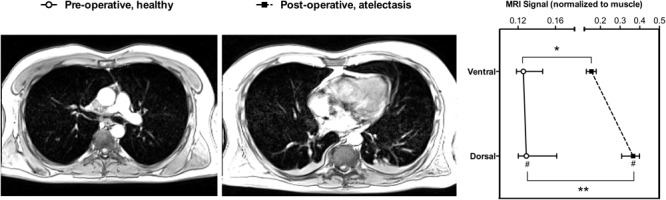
Magnetic resonance imaging quantitative analysis in a representative patient. Pre-operative scan with healthy lungs (Left), and post-operative scan showing atelectasis (Middle). (Right) Shows the lung MRI signal intensity, normalized to muscle signal, of two equally spaced ventro-dorsal ROIs in the pre- and post-operative scans. ^#^Higher compared to the ventral ROI (*p* = 0.031); Significantly higher in the post-compared to the pre-operative scan in the corresponding ROI ^∗^*p* = 0.043, ^∗∗^*p* < 0.0001. MRI, magnetic Resonance Imaging; ROI, region of interest. Symbols are median, error bars the interquartile range.
